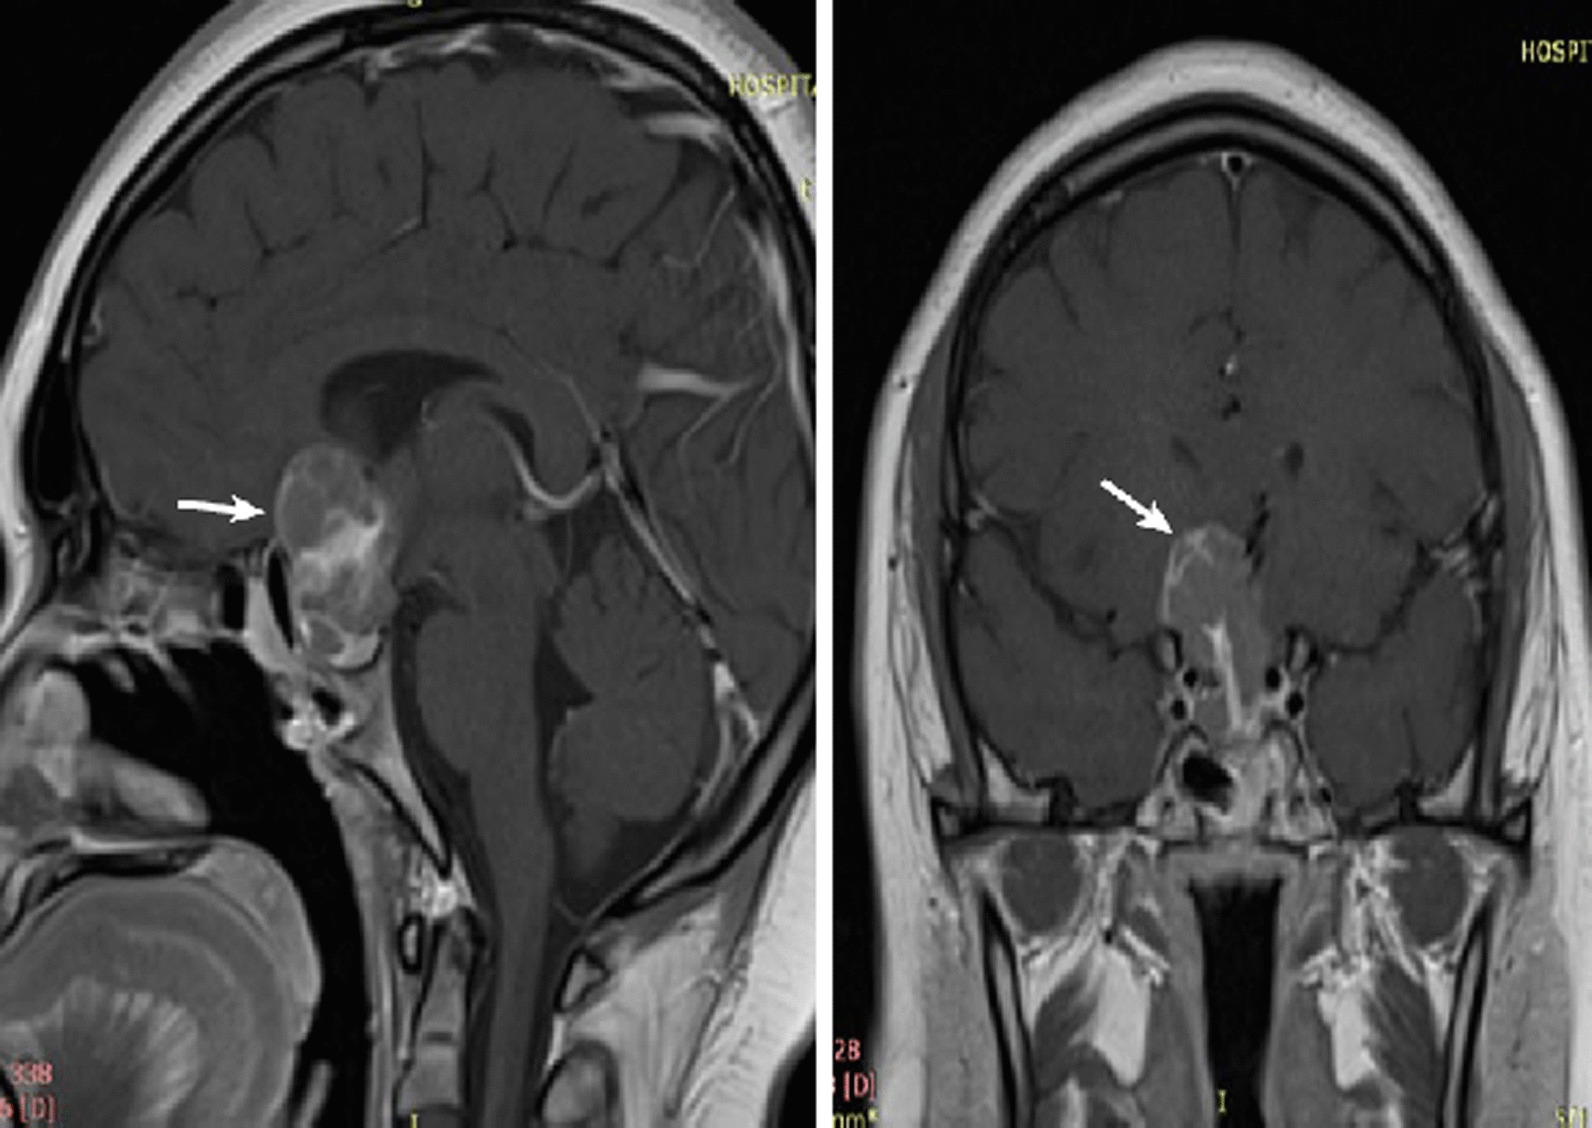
Postoperative sagittal and coronal T1-weighted magnetic resonance imaging pituitary performed after the first transsphenoidal surgery. The magnetic resonance imaging revealed a pituitary lesion with residual mass (size 3.5 × 3.0 × 2.7 cm^3^) seen mainly in the right suprasellar region (white arrow)
